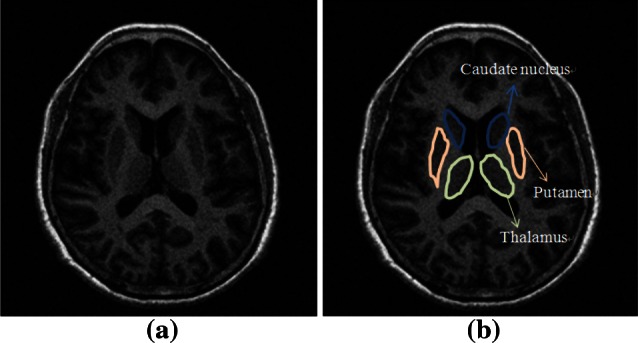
Brain MRI images of a normal and b PD-affected cells
